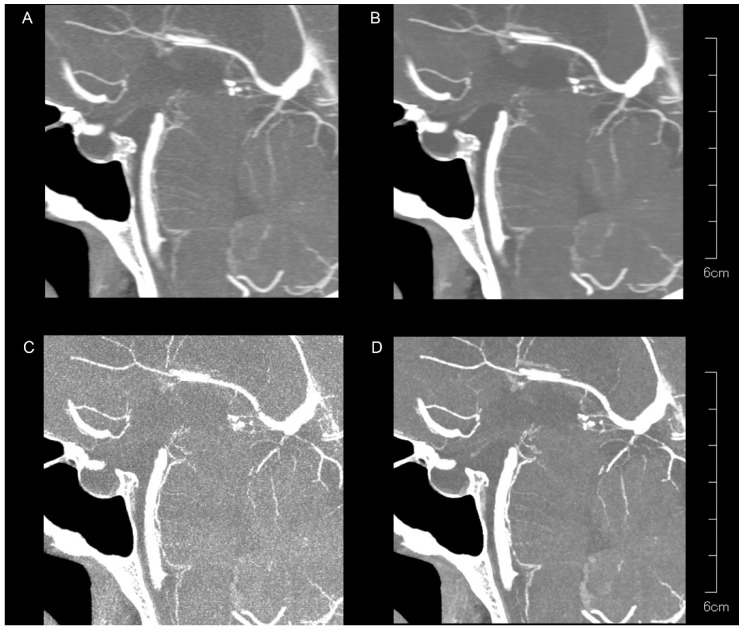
Representative UHR PCD-CTA images with sagittal partial maximum intensity projection for a 71-year-old female, showing kernel Hv40 without QIR (A) and with QIR (B), as well as kernel Hv72 without QIR (C) and with QIR (D) in the PA region. The PAs are less visible compared to the LSAs, with variations in vessel sharpness and image noise among the reconstruction methods.
